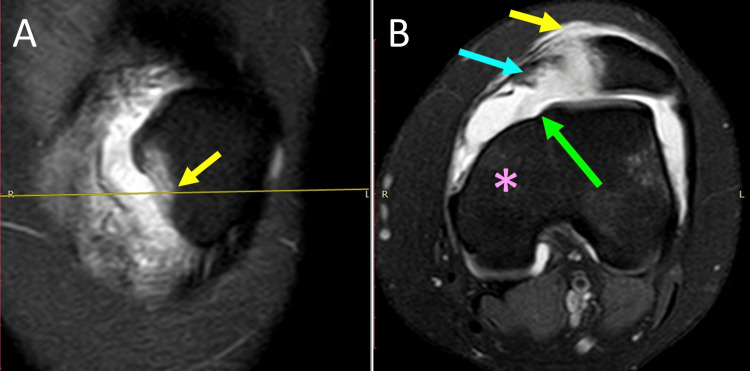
Isolated avulsion fracture of the inferomedial patellar retinaculum after acute left PD in a female adolescent athlete with Dejour-D TD and a hypoplastic medial femoral condyle MRI of the left knee: (A) Frontal plane: the arrow indicates the location of the avulsion fracture at the distal part of the medial patellar border. (B) Axial plane: patellar avulsion fracture (blue arrow), complete avulsion of distal medial restraints from the patella (yellow arrow), Dejour-D TD with a hypoplastic medial femoral condyle (asterisk), and a cliff-type appearance of the trochlea without a trochlear sulcus (green arrow). Figure published under Creative Commons Attribution License CC-BY 3.0 [40].
